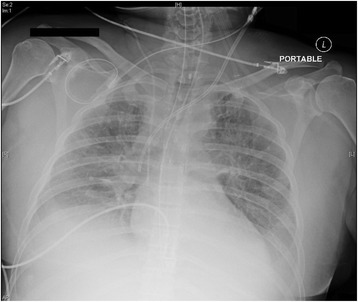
Portable anterior-posterior chest X-ray revealing diffuse bilateral opacities in keeping with pulmonary edema
